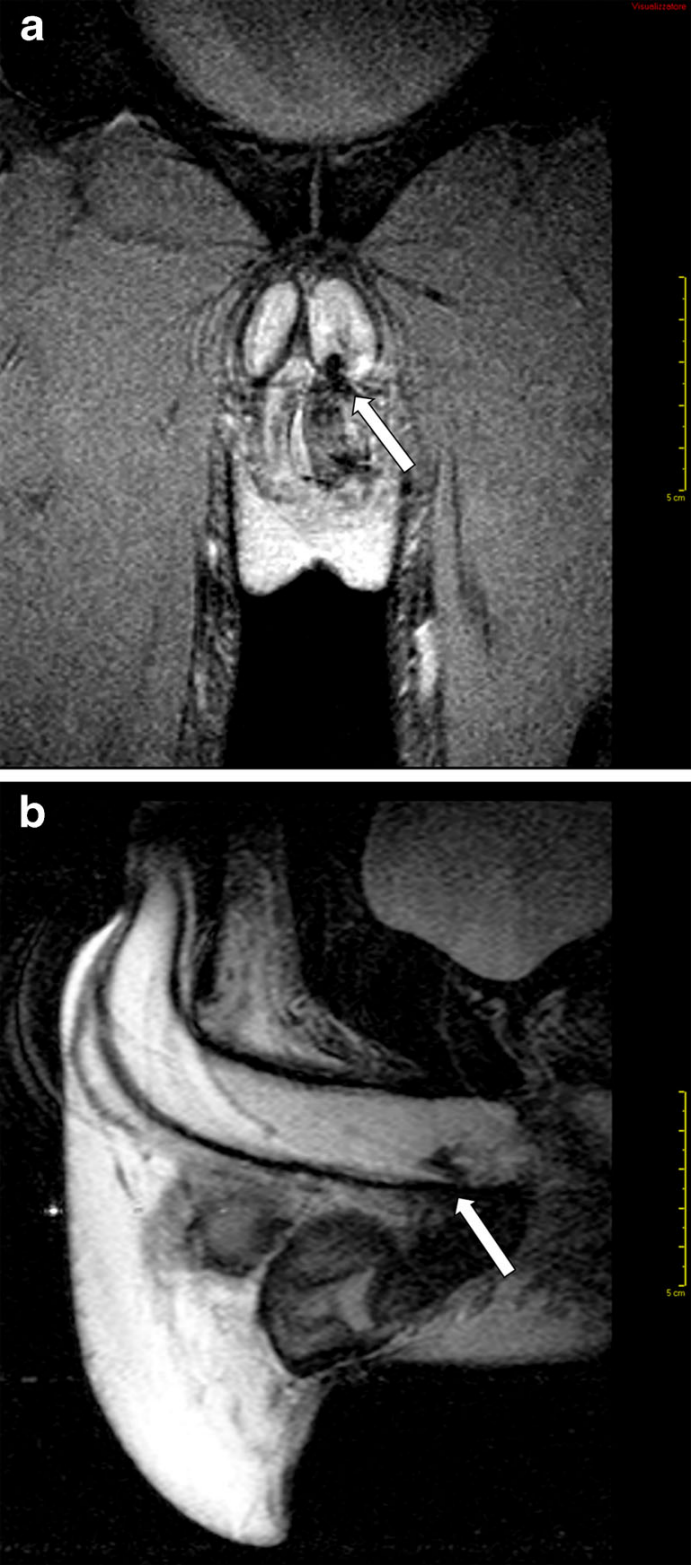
a-b Intrascrotal rupture of the corpus cavernosum (arrow). a T2-weighted FFE sequence, coronal view; b T2-weighted SPIR TSE sequence, sagittal view
